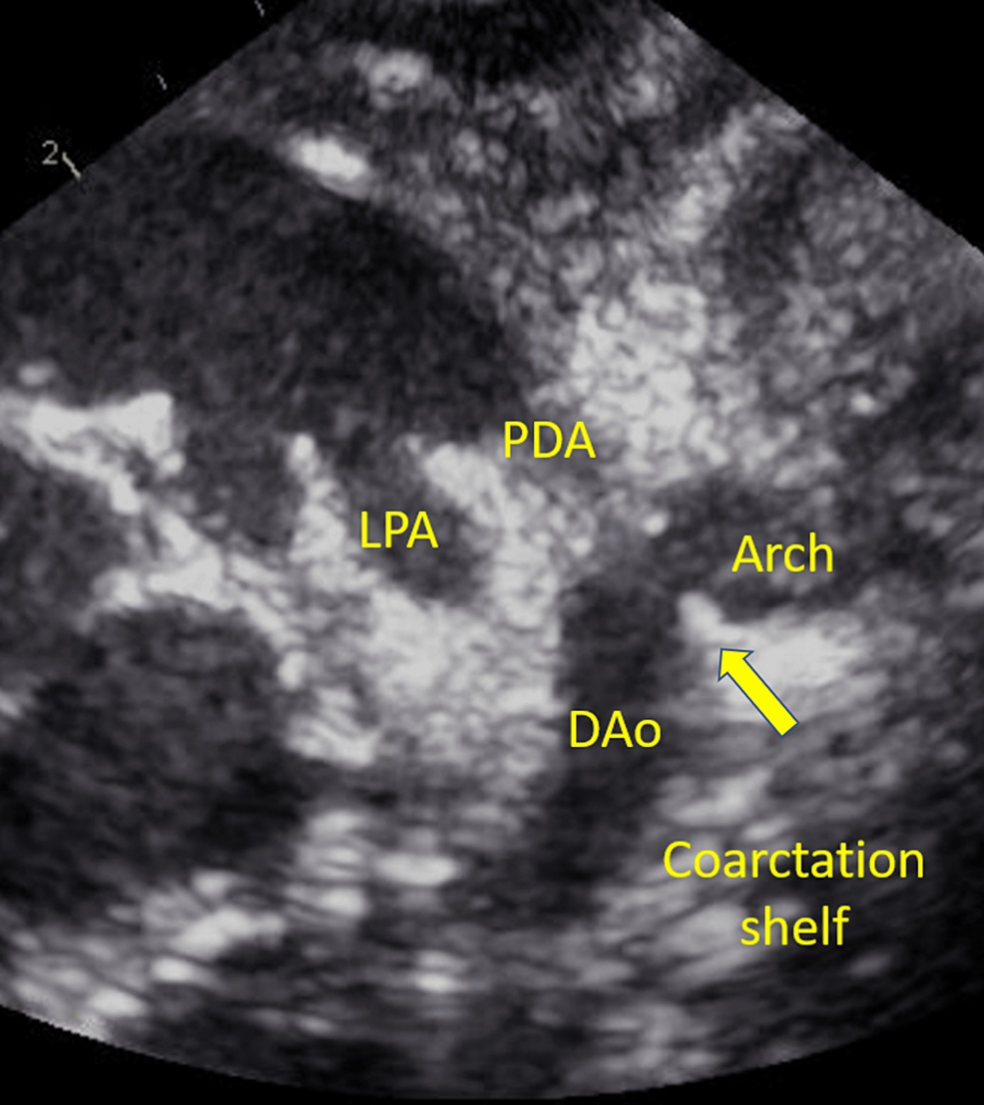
Postnatal echocardiography (arrow) Echocardiography, after DA closed, showed a coarctation shelf on the isthmus. PDA: patent ductus arteriosus, LPA: left pulmonary artery, DAo: descending aorta, DA: ductus arteriosus.
